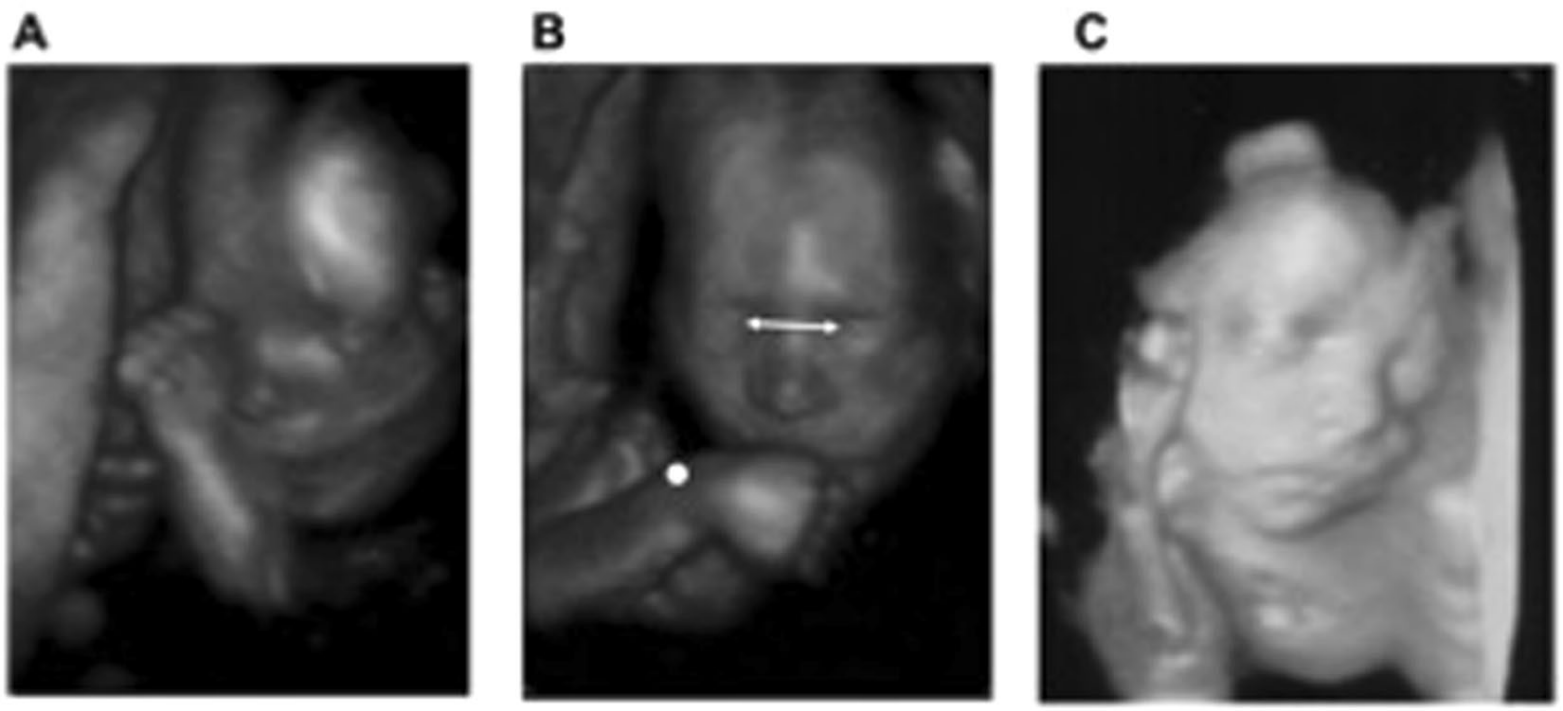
Fetal movements. Frames of ultrasound scans showing the (A) hand to eye, (B) hand to mouth and (C) hand to uterine wall movements. The arrow represents the calibration segment based on the intraocular distance. The white dot represents the digital marker positioned post-hoc.
